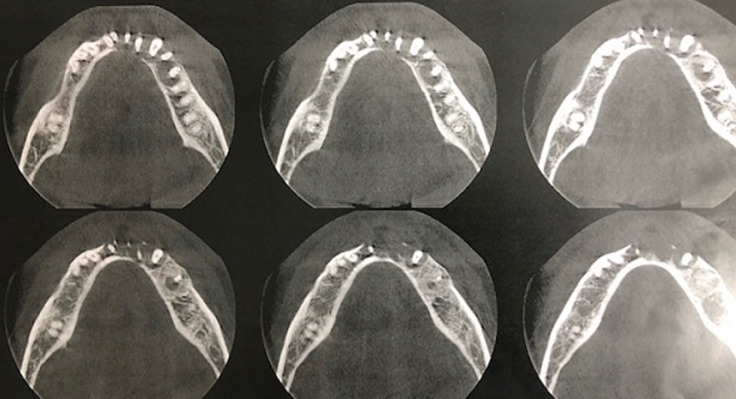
cone beam computed tomography, axial slices: breach of the vestibular cortex and thinning of the lingual cortex
